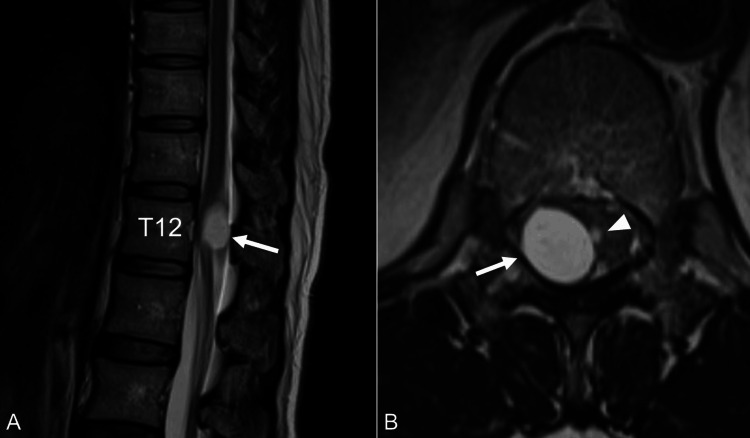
Preoperative magnetic resonance imaging (MRI) of the thoracic spine. (A) Sagittal T2-weighted image showing an intradural extramedullary cyst at the T12 vertebral level (arrow). (B) Axial T2-weighted image demonstrating the cyst (arrow) and a subtle surface irregularity (arrowhead) on the right side of the spinal cord surface.
